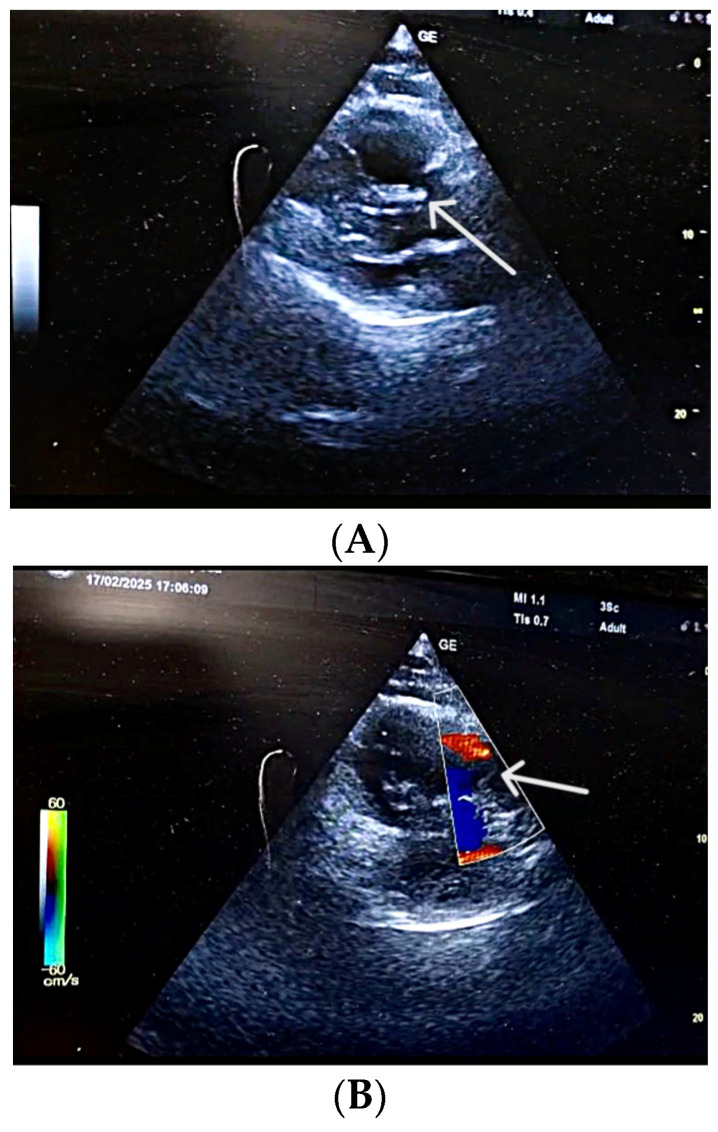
(A) POCUS-TTE performed pre-thrombolysis, showing a markedly dilated right ventricular cavity. Parasternal long-axis view showing acute dilatation of the right ventricle and pulmonary annulus, resulting in incomplete coaptation of the pulmonary valve cusps secondary to an abrupt increase in pulmonary artery pressure (white arrow). (B) POCUS-TTE performed pre-thrombolysis, showing sudden right ventricular pressure overload. Parasternal short-axis view showing the main pulmonary artery trunk with functional pulmonary regurgitation (white arrow).
